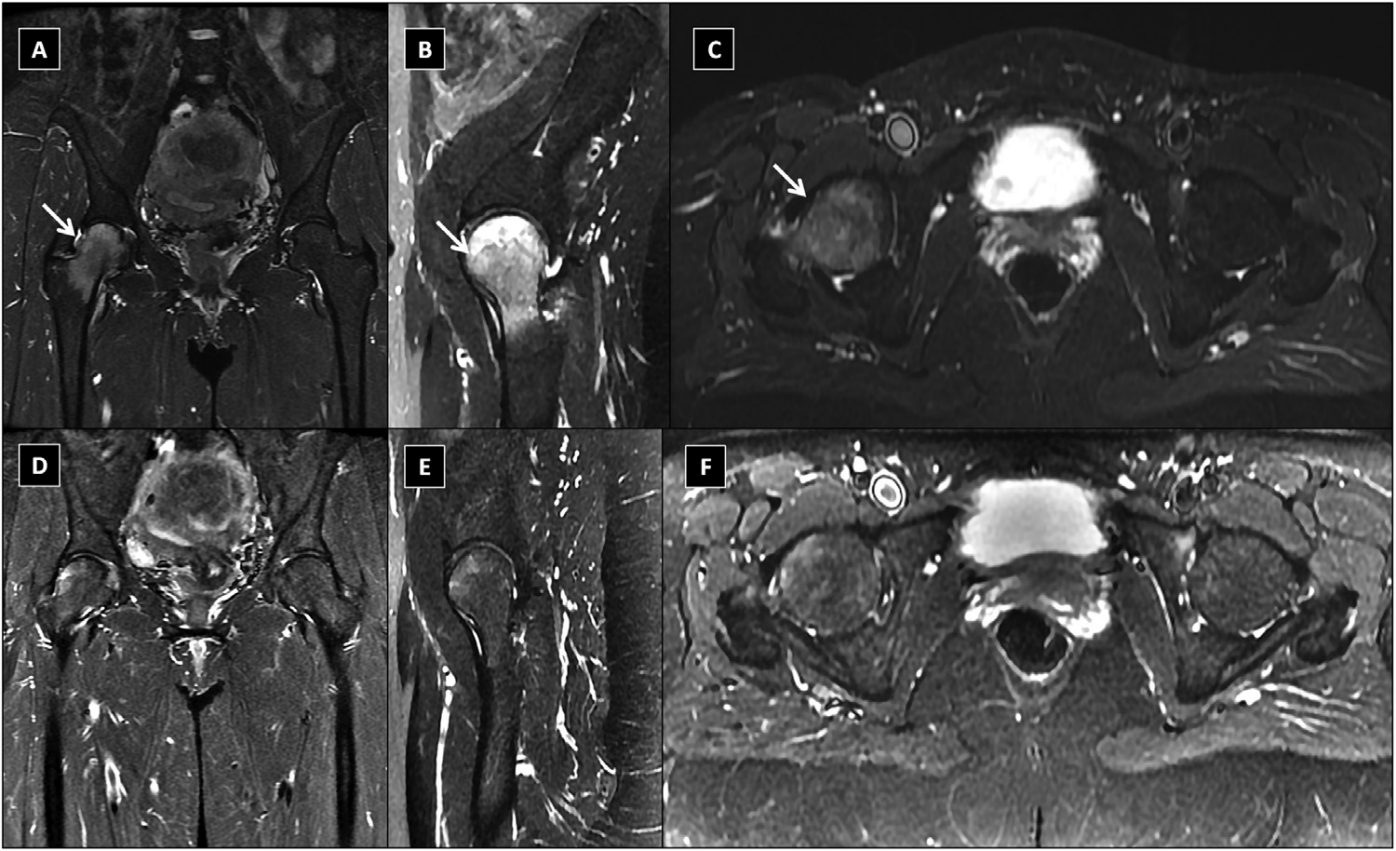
Initial magnetic resonance imaging (MRI) study for both hip joints: (a): coronal; (b) Sagittal; (c) axial Short tau inversion recovery (STIR) sequences: showing extensive bone marrow edema (BME) affecting the right femoral head, right femoral neck and extending to the intertrochanteric region: Follow-up MRI study showing the corresponding STIR sequences (d, e, –f) after 3 months of treatment with Alendronate showing almost total regression of the right femoral head neck marrow edema. The articular cartilages are preserved with no femoral head structural collapse could be detected.
